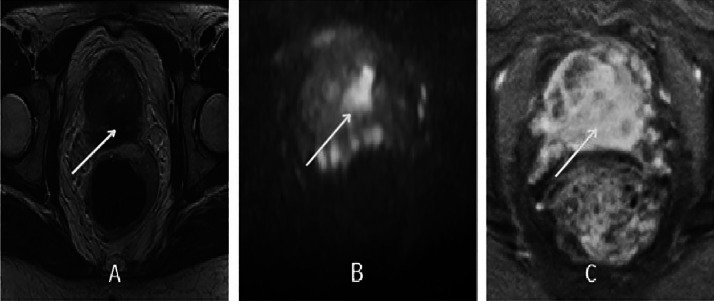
Axial-section MRI images in T2 (A), Diffusion (B) and T1 sequence after gadolinium injection (C), showing a tumor patch in the medio-prostatic peripheral zone in T2 hyposignal and Diffusion hyper signal with significant gadolinium uptake after injection (white arrows).
